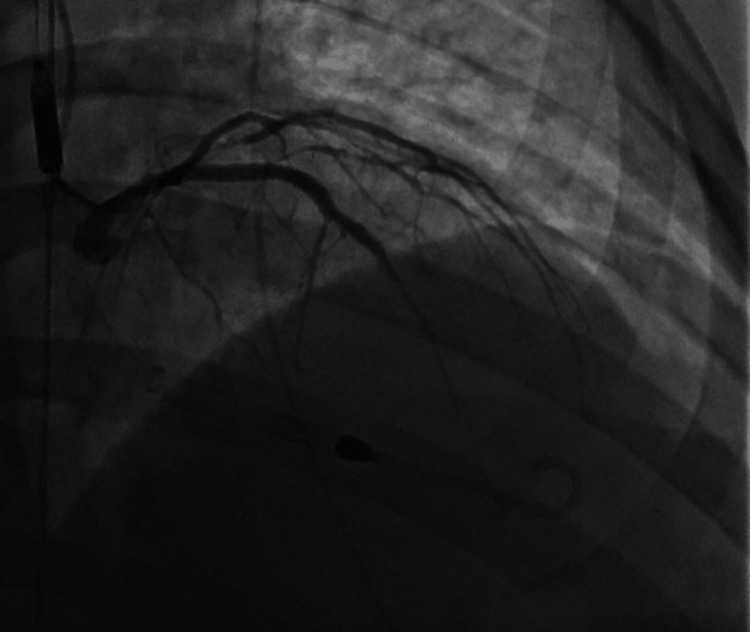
Left heart catheterization Follow-up coronary angiography after intravascular ultrasound-guided repeat percutaneous coronary intervention demonstrates restoration of coronary patency with improved distal flow in the left anterior descending and obtuse marginal arteries. Mechanical circulatory support with an Impella CP^®^ device was initiated due to markedly elevated left ventricular end-diastolic pressure and hemodynamic instability. The device is visualized within the left ventricle, providing temporary circulatory support during high-risk revascularization.
